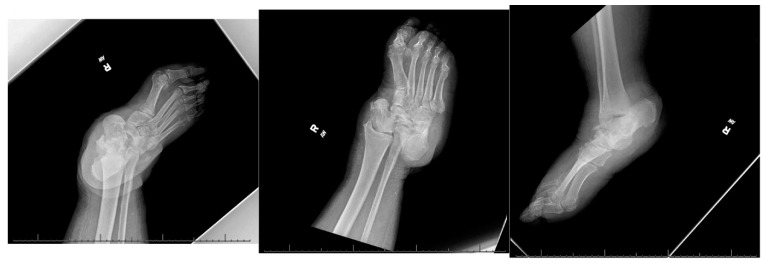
Preoperative radiographic images.
